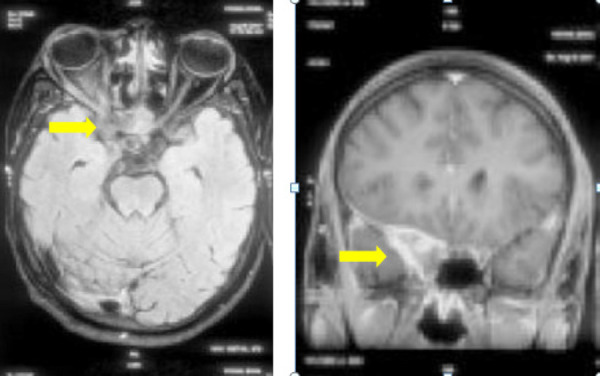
MRI T1W axial and Gad T1W coronal images showing the lesion enhancement in post gadolinium study.
